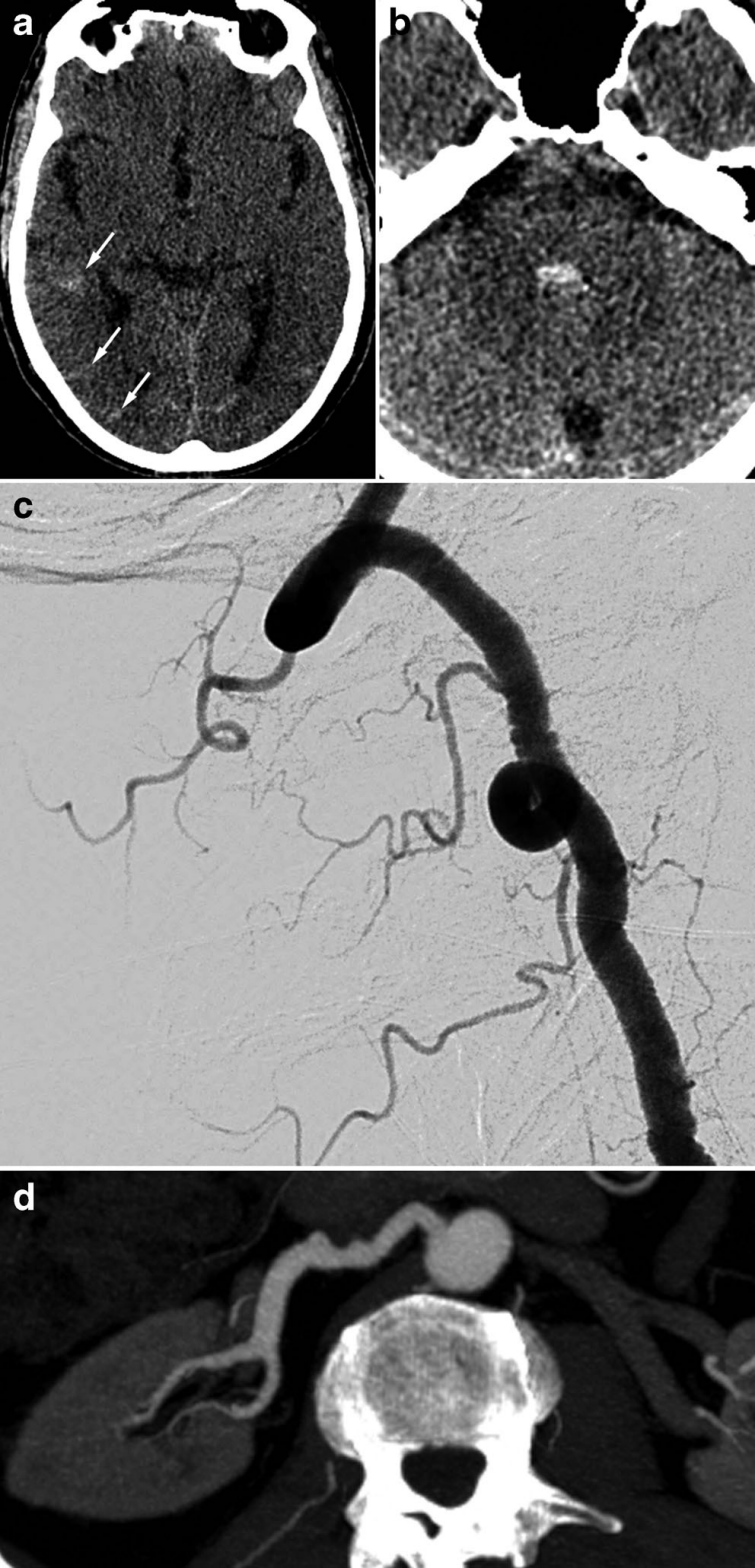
Radiologic findings in a 65-year-old male patient with SAH of the posterior fossa. The CTA shows no particular signs. The arteriography found typical FMD lesions in the right vertebral artery. The renal CT shows ectasia of the right renal artery termination. a and b Cerebral non-enhanced CT showing right temporo-occipital SAH (*white arrows*) associated with intraventricular haemorrhage (V4). c Right vertebral angiogram showing the typical string-of-beads aspect. d Renal CTA, curved reconstructions of the right renal artery showing ectasia of the distal part of the right renal artery
